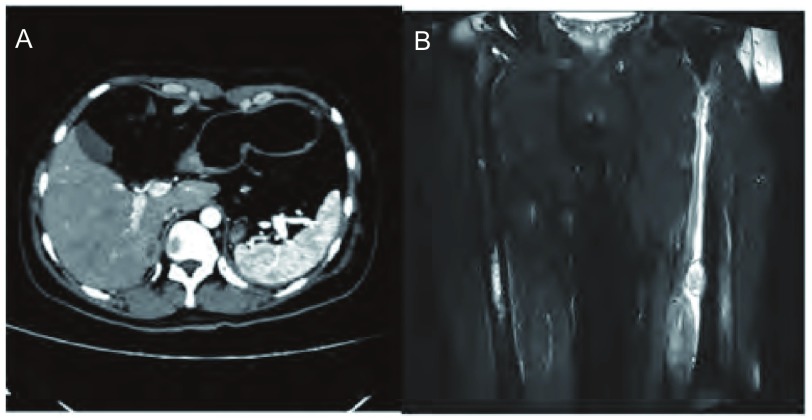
A：肾上腺增强CT提示：双侧肾上腺可见梭形异常强化影，右侧大小为2.2 cm×1.3 cm，左侧大小为1.3 cm×0.8 cm；B：磁共振左大腿提示：左侧股骨中下部内前方股中间肌下方见结节状不均匀等T1长T2信号影，大小约1.5 cm×2.3 cm×3.2 cm，所示右侧股骨上段、中下段骨髓腔内见斑片状长T1长T2信号影. A: Adrenal enhanced CT prompt fusiform enhancement in inside limbs of bilateral adrenal with size of 2.2 cm× 1.3 cm in the right and 1.3 cm×0.8 cm in the left; B: magnetic resonance of left thigh show nodosity uneven nodular T1 long T2 signal below vastus intermedius muscle in front of the inside part of the left femurl with size of 1.5 cm×2.3 cm×3.2 cm, and show pachy long T1 long T2 signal inside the upper and middle-lower bone marrow cavity.
